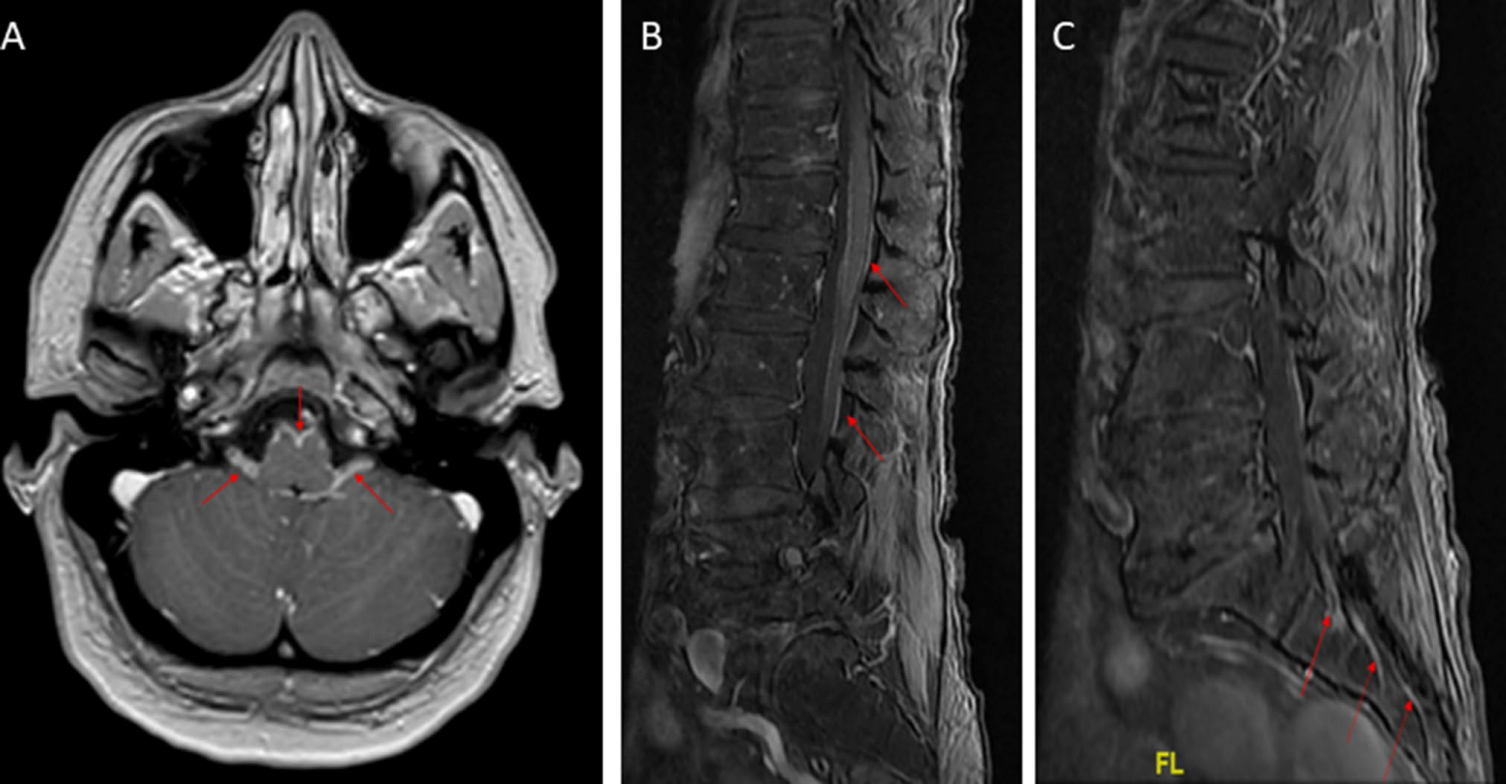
Brain and spinal cord MRI features of patients with CSF GFAP antibodies and PNS involvement. Brain MRI of a patient with glial fibrillary acidic protein (GFAP) antibodies showing brainstem leptomeningeal and acoustic-facial bundle contrast enhancement (A, red arrows). Spinal cord MRI of a patient with GFAP antibodies showing medullar leptomeningeal and root contrast enhancement (B, C, red arrows)
